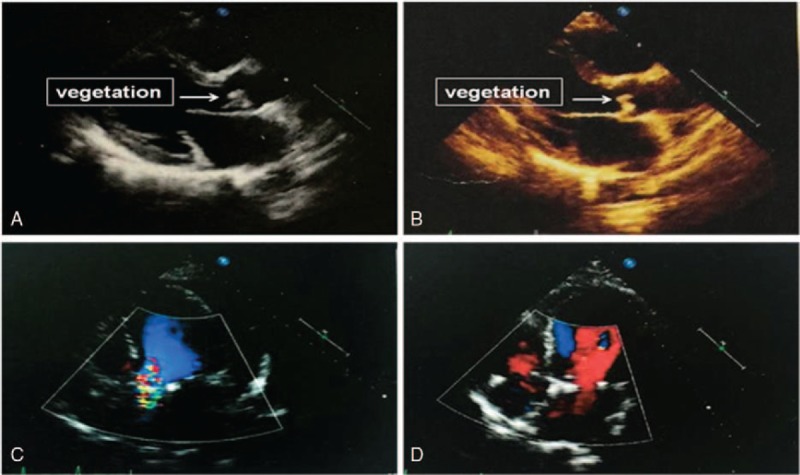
Echocardiography images during hospitalization. (A and B) Echocardiography images obtained on April 10, 2015 and May 7, 2015, where arrow point at revealed a well-shaped vegetation attached to the aortic valve. (C and D) The images obtained on June 4, 2015, 1 week after surgery, and September 08, showed no left-to-right atrial shunt after surgery.
